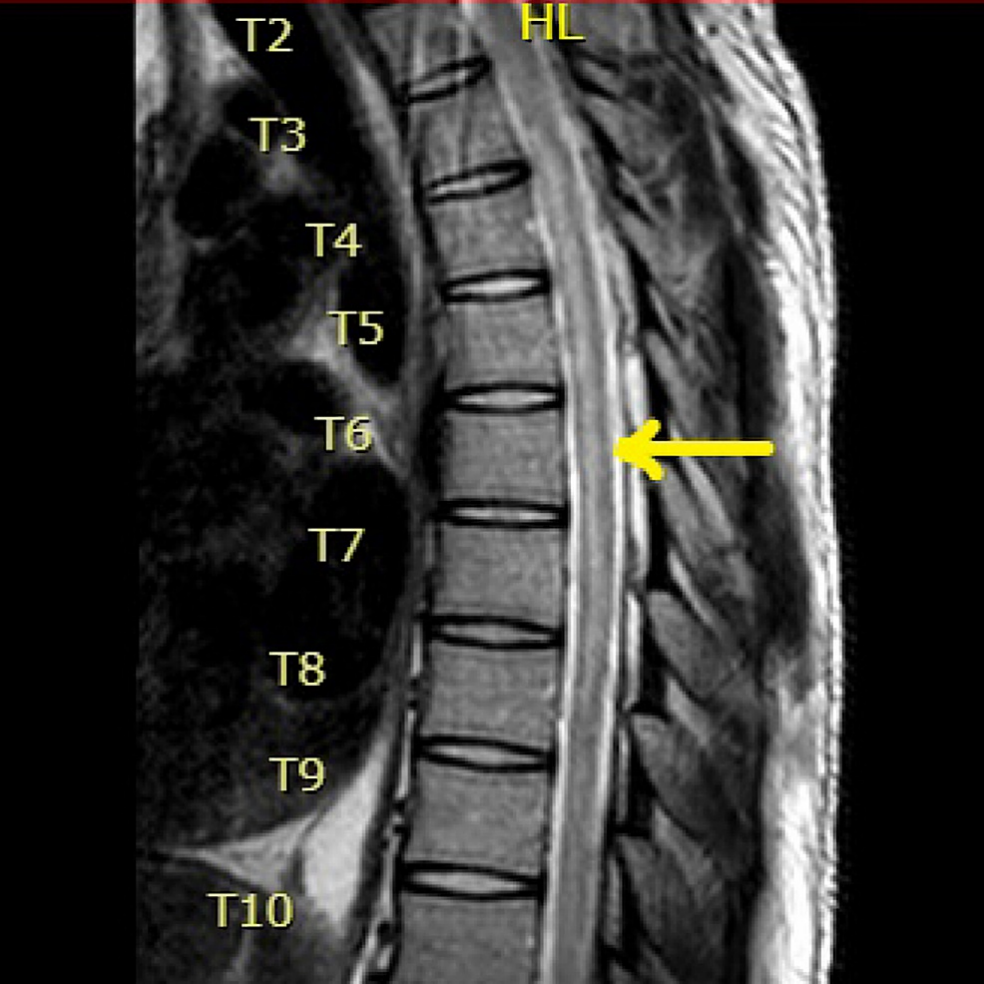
MRI of thoracic spine shows abnormal T2 signaling spanning from T2-T8, highly suspicious for an acute spinal cord infarction (focal cord swelling and "pencil-like" hyperintensities on T2-weighted images).
